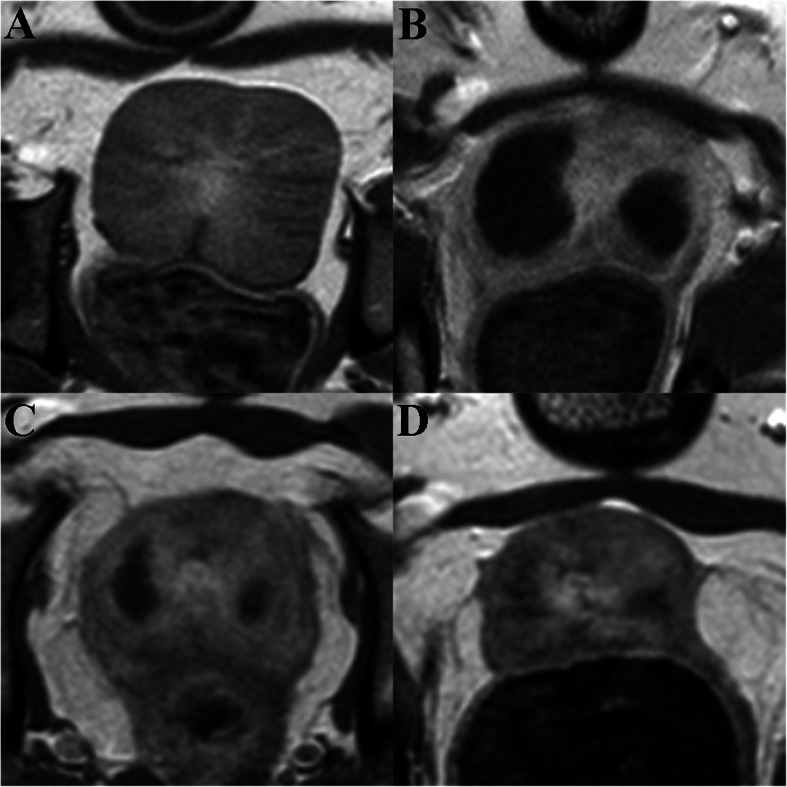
Contrast enhanced axial T1-weighted. MR images show​ the prostate immediately before PAE (a), 1 week (b), 2 weeks (c), and 1 month (d) after PAE. The infarct lesions lacking of enhancement gradually decrease in size with time and the prostate shrinks substantially at 1-month follow-up (d)
